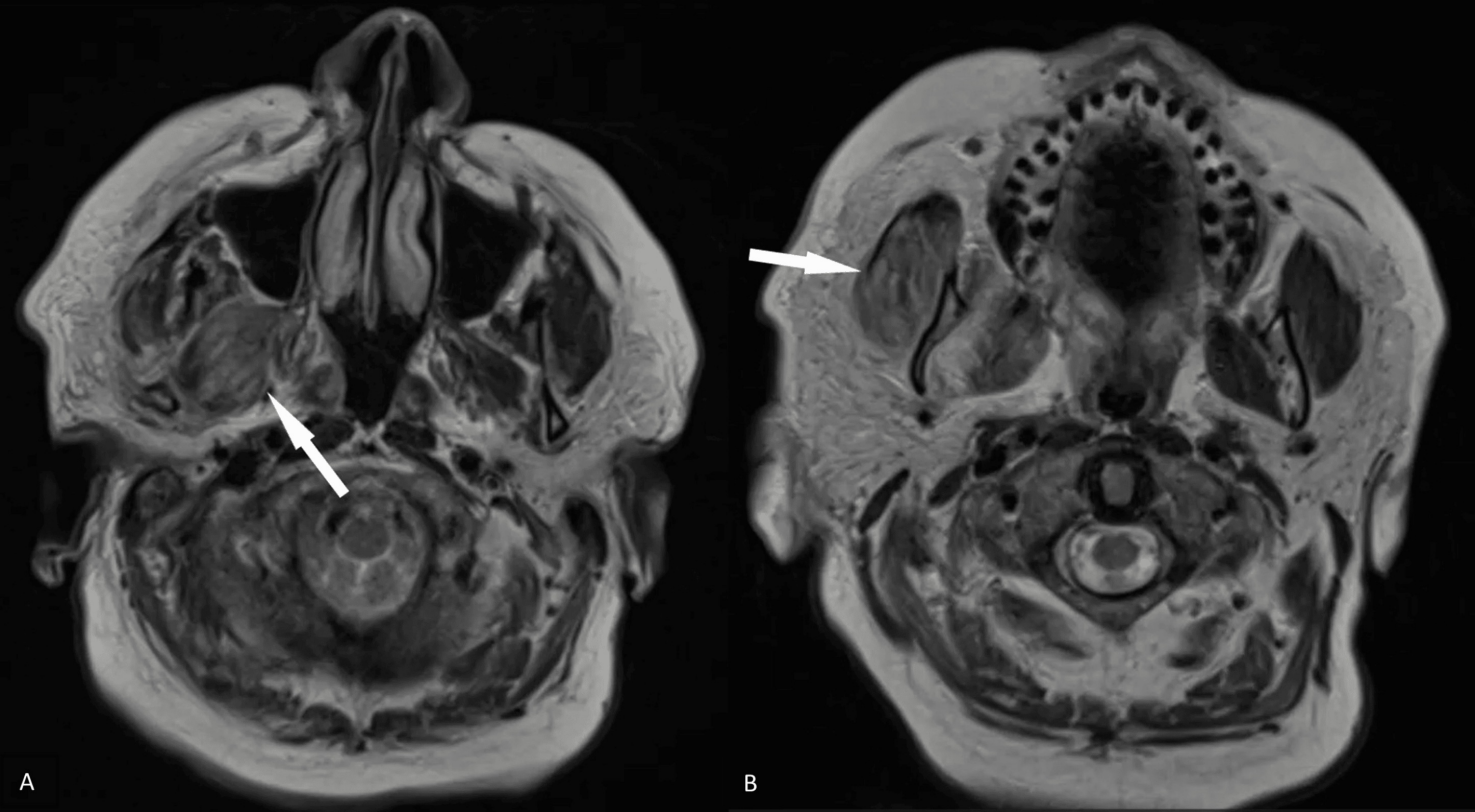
MRI images of the brain showing findings of right masticator space cellulitis. Axial T2-weighted images demonstrate asymmetric enlargement and increased T2 signal intensity within the muscles of the right masticator space, namely the medial pterygoid (white arrow, A) and masseter muscles (white arrow, B) indicative of right masticator space cellulitis.
